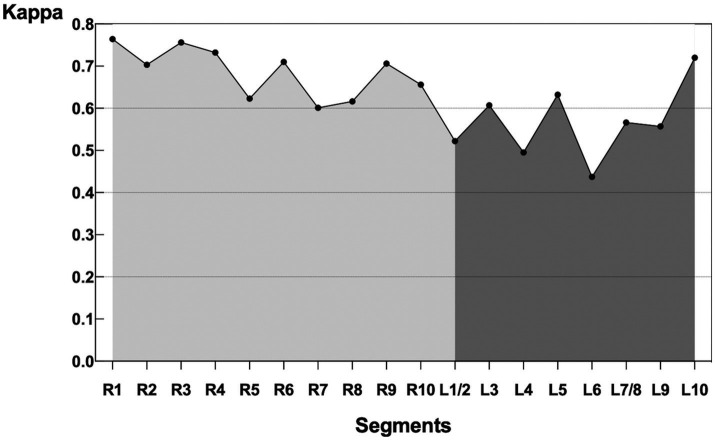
Kappa of each segment comparing PW-PREFUL MRI with V/Q SPECT/CT. For each segment, the level of agreement using the classification scoring system (detecting absent, subsegmental, and segmental hypoperfusion) had a kappa range from 0.44 in the superior segment (L6) of the left lung up to 0.76 in the apical segment (R1) of the right lung. MRI, Magnetic resonance imaging; PREFUL, phase-resolved functional lung; PW, perfusion-weighted; V/Q SPECT/CT, ventilation/perfusion single-photon emission computed tomography/computed tomography.
